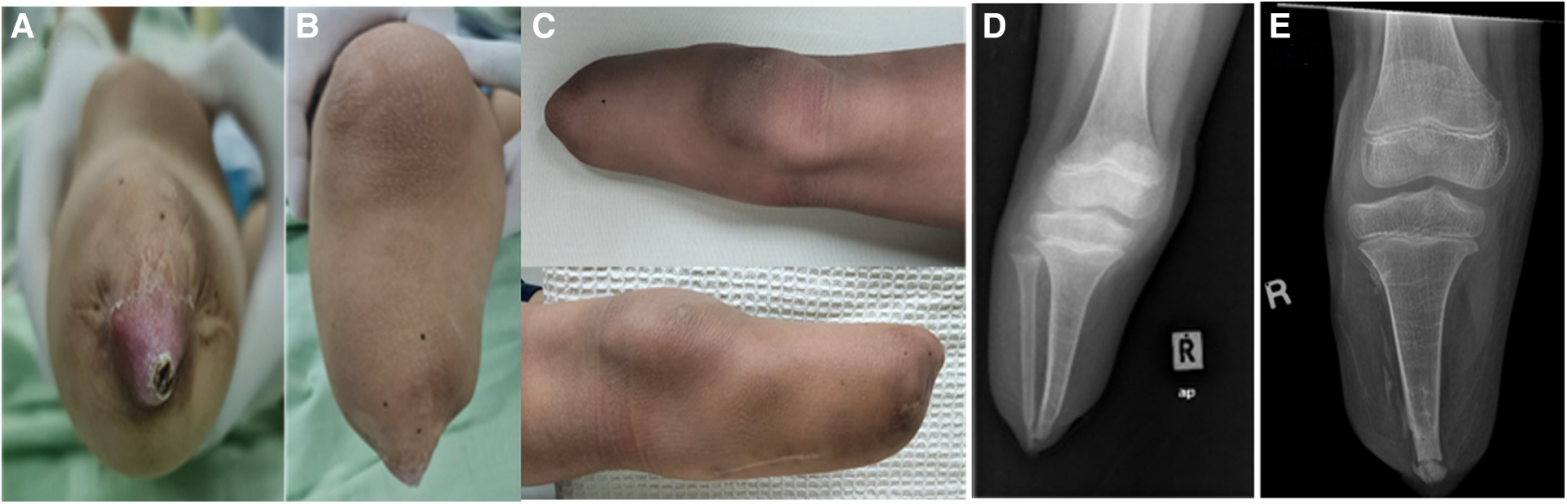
(A) and (B) show ulceration and bony prominence over the right tibial stump prior to the operation. (C) The right tibia stump without any significant osseous growth after 2 years post-operation. (D) The pre-operative plain radiographs revealing excessive osseous growth over the distal tibia and fibula, with the growth almost penetrating through the soft tissue. (E) exhibits post-operative plain radiographs taken 2 years after the surgery, demonstrating the union of the fibula with the distal tibia and the absence of excessive osseous growth.
